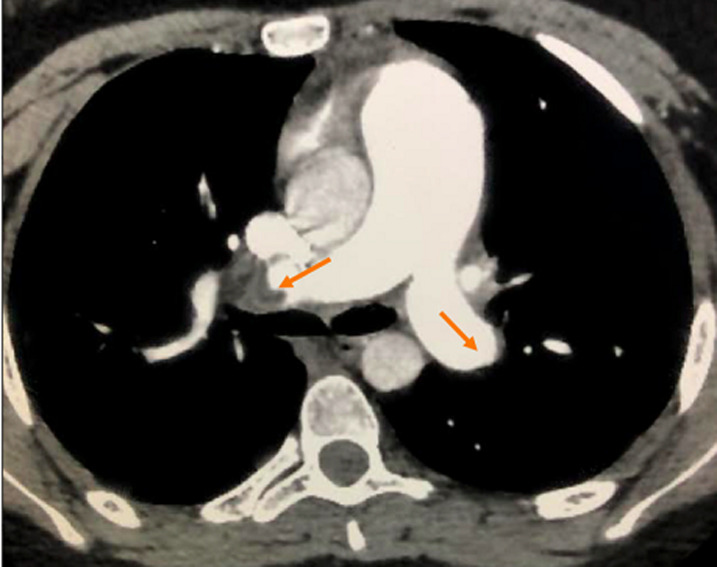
computed tomography angiogram demonstrates bilateral filling defects in the main pulmonary arteries (red arrows)
